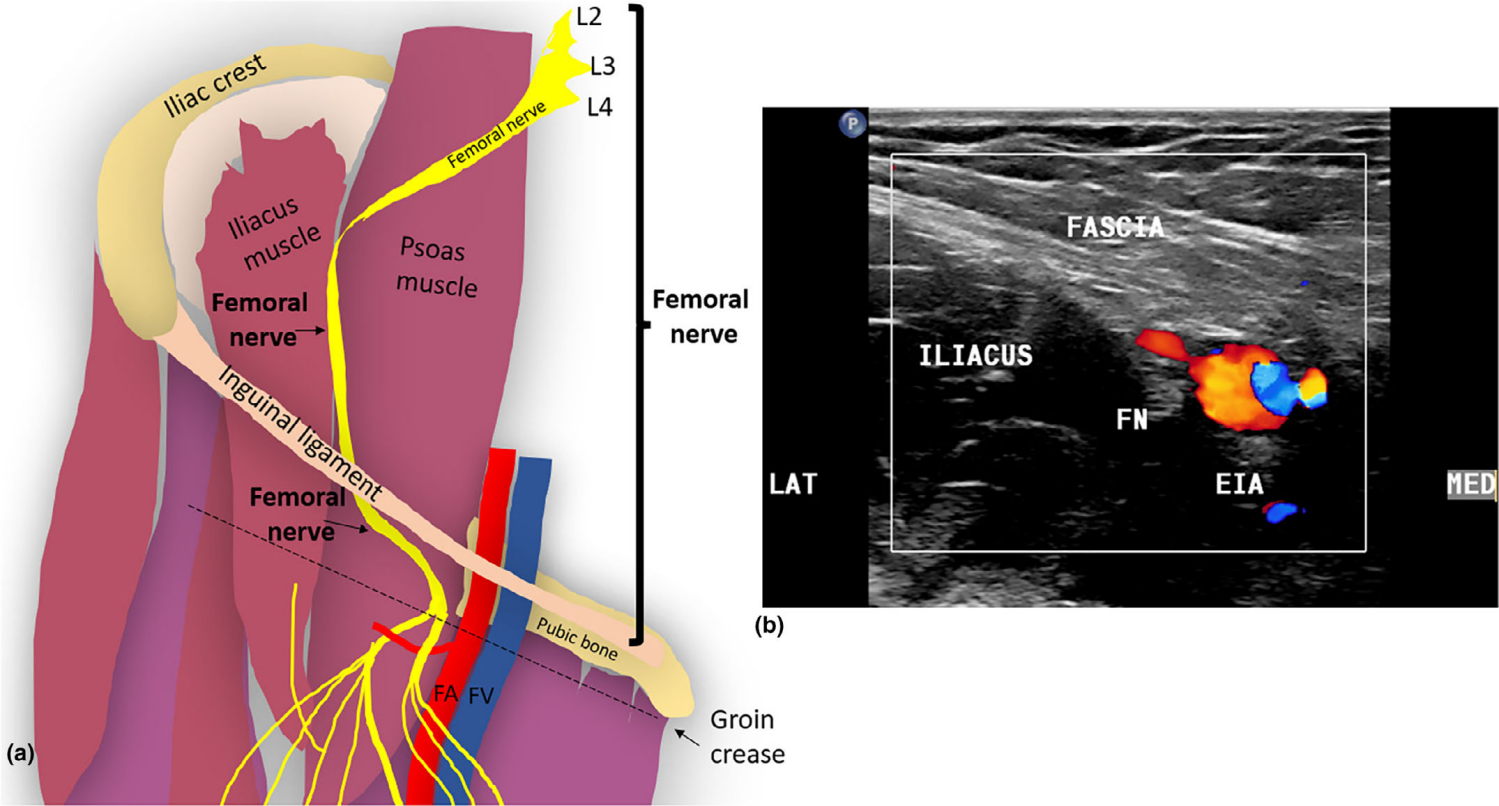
(a) Course of the right femoral nerve through the pelvis. (b) Transverse sonographic image of the right femoral nerve (FN), deep to the fascia iliaca, lateral to the external iliac artery (EIA). FA, femoral artery; FV, femoral vein; LAT, lateral aspect of the image; MED, medial aspect of the image.
